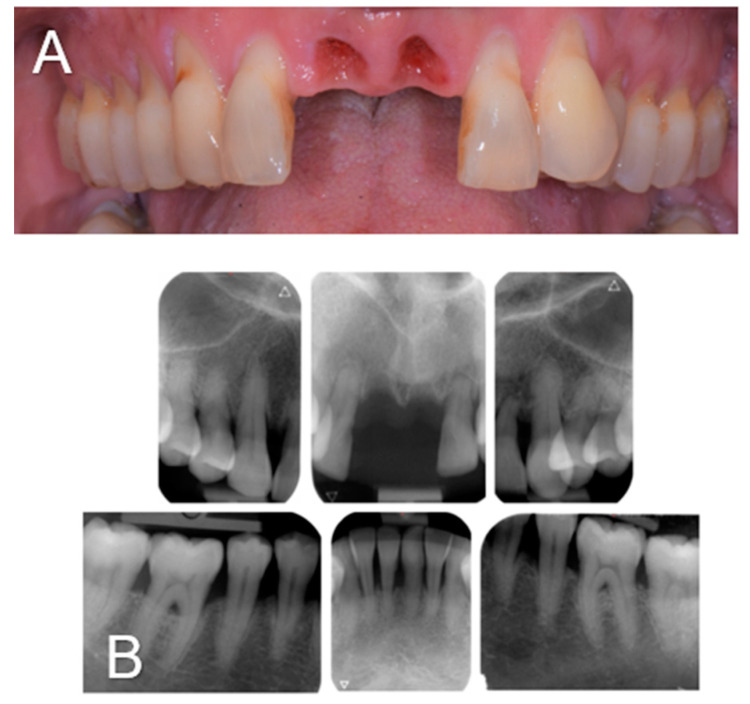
Clinical view (A) and intra-oral radiographs (B) showing the initial clinical status after dental avulsions due to endotracheal intubation as well as the severe chronic periodontal disease.
